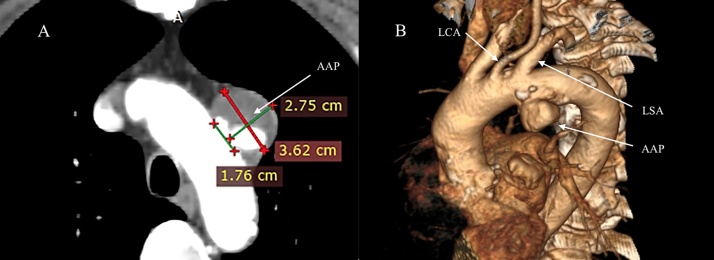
Preoperative contrast enhanced CT scan: (A) CT scan showing the AAP at the level of the aortic arch, (B) 3D CT-scan showing the AAP, with the neck present just caudally from LSA on the lesser aortic arch curvature. (AAP - aortic arch pseudoaneurysm, LSA - left subclavian artery).
